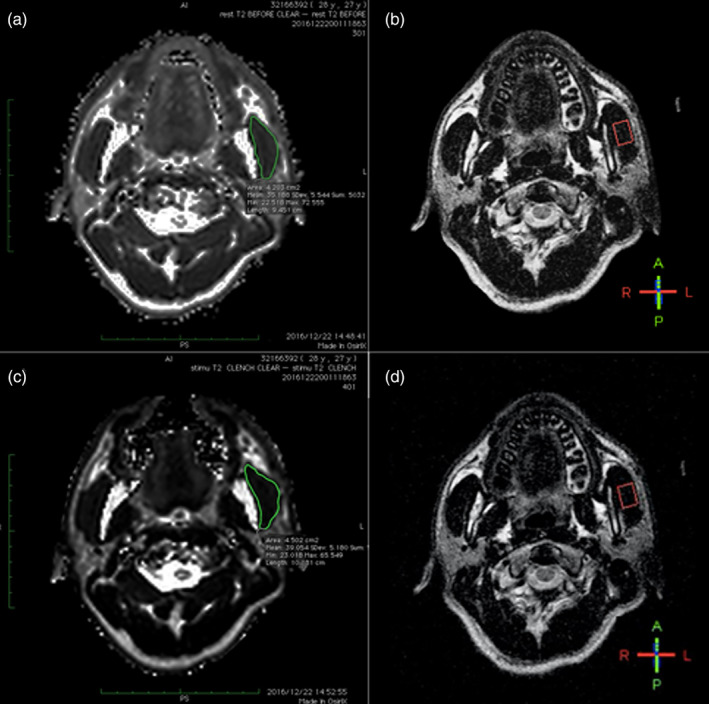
(a, c) Representative axial plane T2‐weighted MR images obtained at the midbelly of the masseter muscle at rest (a) and after clenching (c). Measurement of the T2 value and cross‐sectional area of the masseter muscle. The region of interest (ROI) on a defined axial slice (green line) was measured. (b, d) the position of the ROI on ^31^P‐MRS for the masseter muscle was set so as to obtain as large a pixel size as possible (red line)
